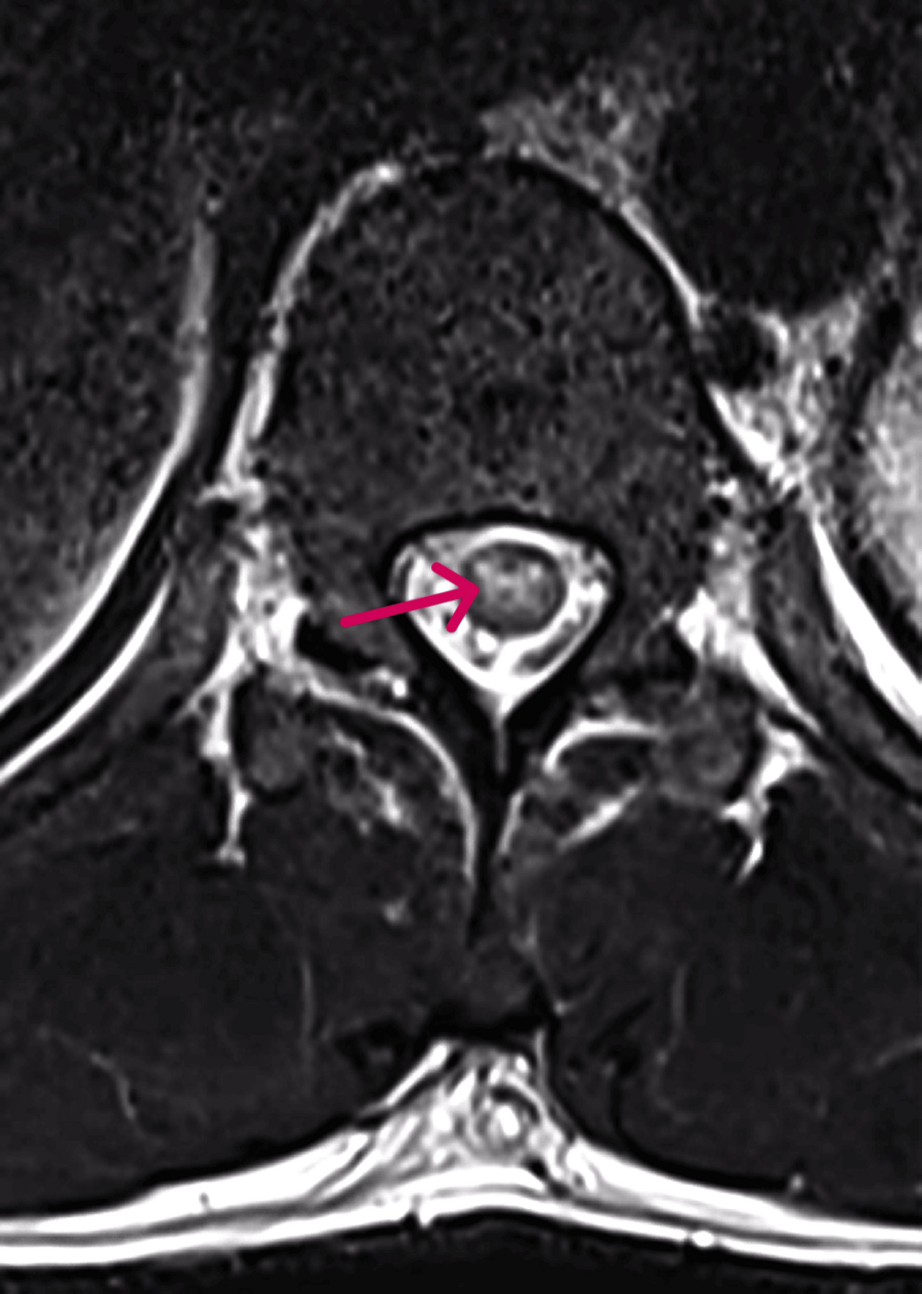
T2 weighted MRI of axial spine indicating pathology restricted to central gray matter within the spinal cord
